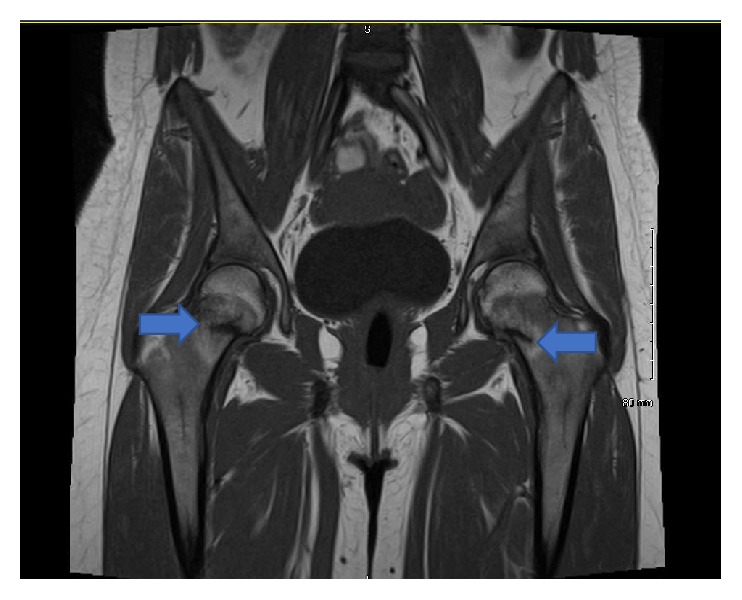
MRI Pelvis showing bilateral stress insufficiency fractures of the hip, femoral neck*. On the right, the transcervical low signal line extends to the superior lateral cortex and is associated with marrow edema, suggestive of a nondisplaced transcervical fracture.
